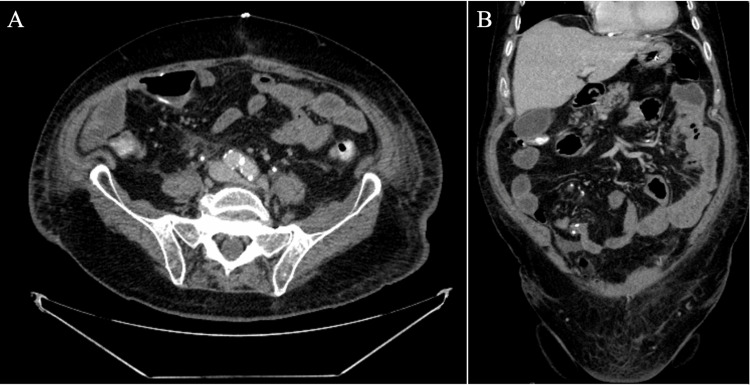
CTAP with IV contrast on post-op day 10 demonstrating resolution of SBO and progression of enteric contrast into the rectum (A: Axial, B: Coronal). CTAP: CT Abdomen and Pelvis; SBO: Small Bowel Obstruction.
